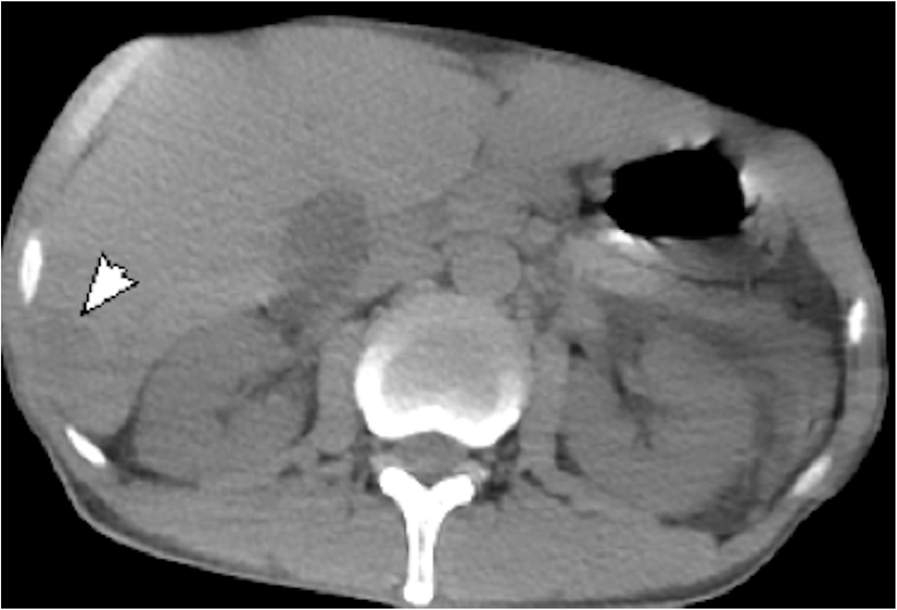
Abdominal CT scan demonstrating an area of abnormal attenuation measuring 25 mm × 19 mm in the right lobe of the liver
